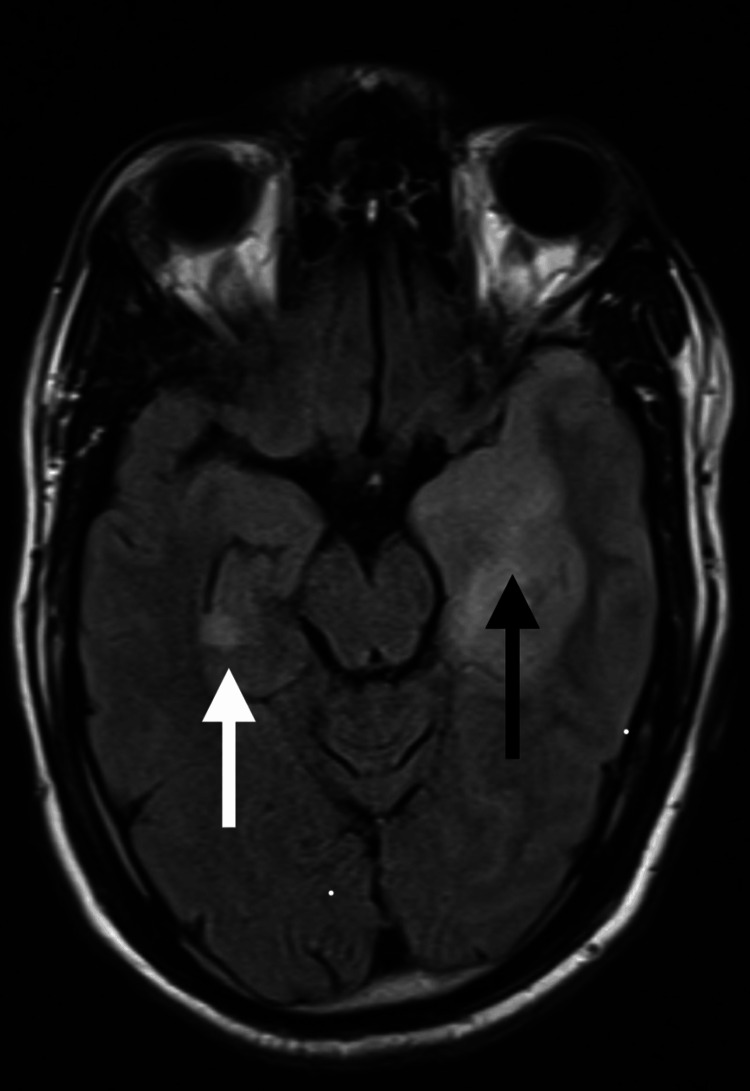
MRI brain axial FLAIR image taken on January 24, 2024, showing left mesial temporal hyperintensity with obliteration of the temporal horn and mild compression of the midbrain (indicated by black arrow) and right hippocampal hyperintensity (indicated by white arrow). FLAIR: Fluid-attenuated inversion recovery.
